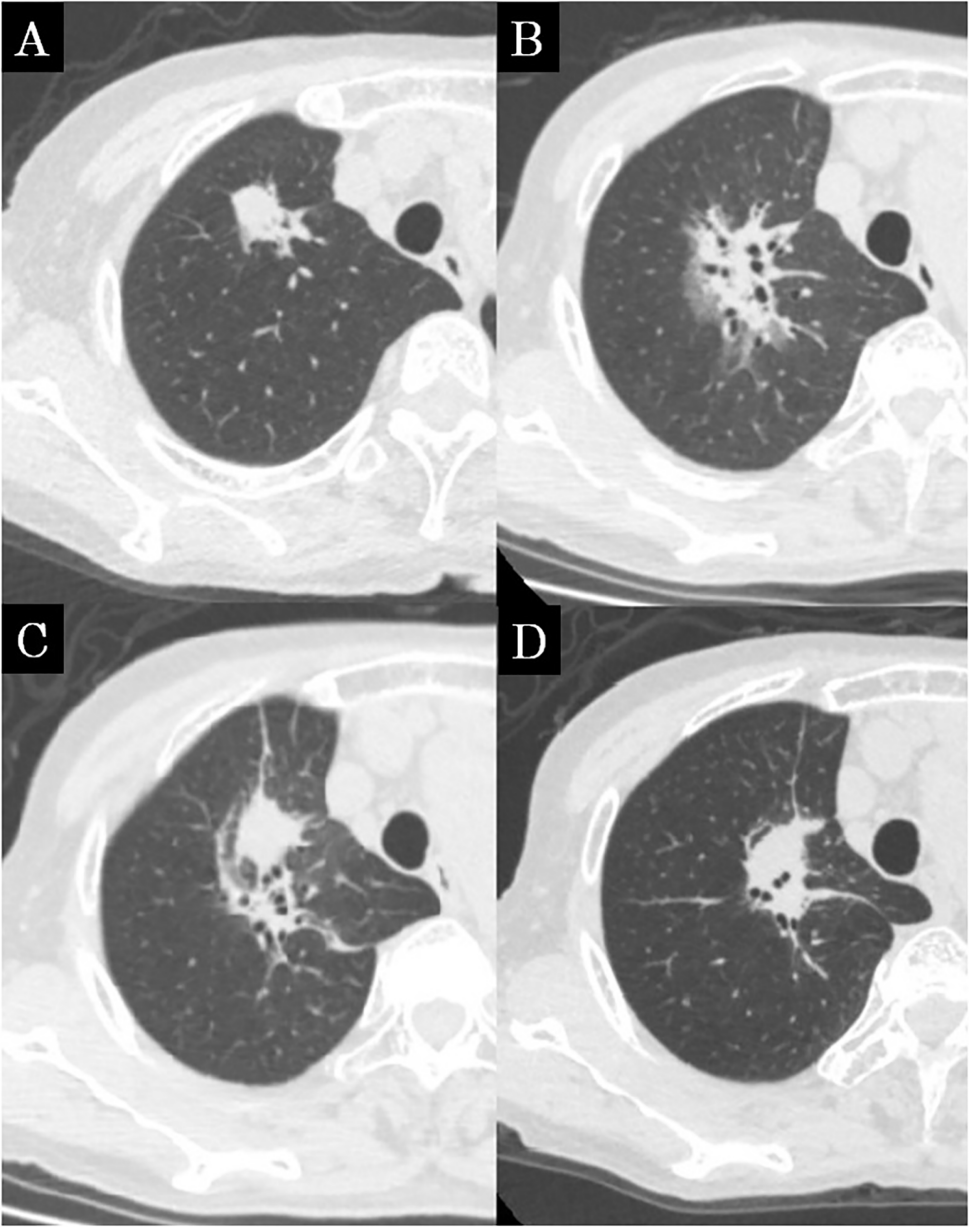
Concordance CT appearance, case 3. (a) Before SBRT, the lung tumor was located in the right upper lobe. (b) Patchy areas of hazy and consolidation are seen; this shadow was diagnosed as patchy consolidation and GGO at 5 months after SBRT. (c) The shadow forms a focal consolidation; this was diagnosed as a mass-like pattern at 17 months after SBRT. (d) The shadow shrank, but a mass-like shadow remained at 35 months after SBRT.
